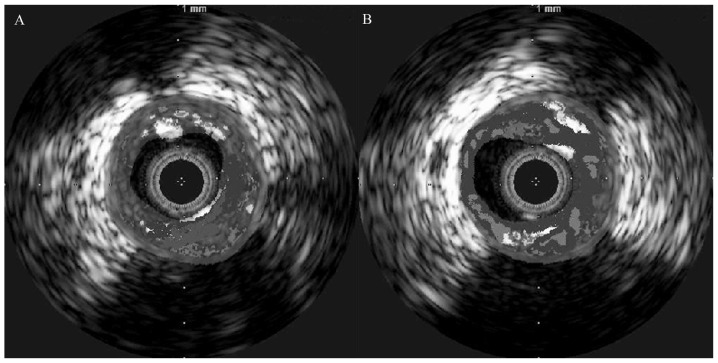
Intravascular ultrasound (IVUS) of two different plaques. (A) Unstable plaque. (B) Stable plaque.
